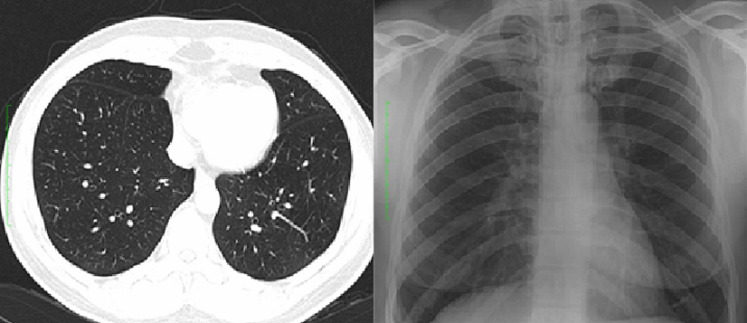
chest radiograph and CT images of case 1 on the 5^th^ and 19^th^ days of onset of symptoms respectively showing no evidence of pneumonia
